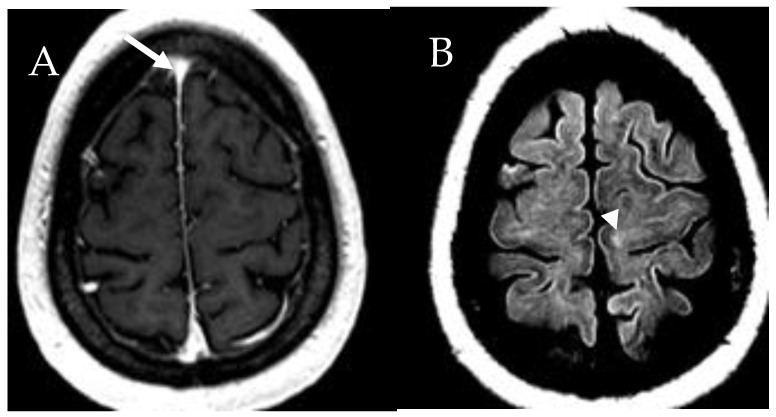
A 55-year-old male patient with systemic mastocytosis. (A) Axial postcontrast T1-weighted image demonstrates punctate focus of enhancement within the right frontal lobe cortex (arrow). (B) Focal T2 hyperintensity in the subcortical left temporal lobe (arrowhead).
